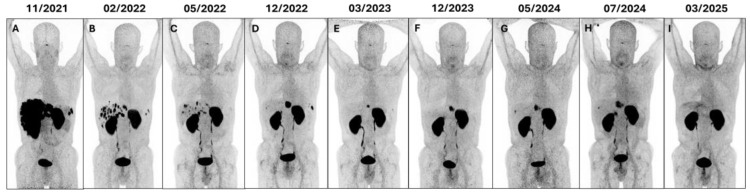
MIP sequence of [^68^Ga]Ga-DOTA-LM3 PET/CT scans of a 52-year-old patient diagnosed in 2014 with well-differentiated, functioning NEN of the pancreas G3 (Ki67 52%). He underwent pancreaticoduodenectomy and, due to hepatic and peritoneal lesions, received chemotherapy (2014), liver metastases resection (2018), 4 cycles of [^177^Lu]Lu-Oxodotreotide (Lutathera^®^, 2020), Sunitinib, Everolimus, Pembrolizumab, and somatostatin analogues (2021). Restaging with Gallium-68-labeled SSTR antagonist PET/CT ((A); 11/2021) showed massive progression with innumerable liver metastases and increased chromogranin A (>2000 µg/L), prompting two cycles of [^177^Lu]Lu-DOTA-LM3 PRRT (11/2021–02/2022), achieving partial remission (B,C). He also received immunotherapy with Nivolumab. Peritoneal recurrence in the upper abdomen led to two more cycles of [^177^Lu]Lu-DOTA-LM3 PRRT (12/2022–03/2023), resulting in minimal residual disease at [^68^Ga]Ga-DOTA-LM3 PET/CT (D,E). Subsequent [^68^Ga]Ga-DOTA-LM3 PET/CT scans (F–H) documented abdominal progression with increased chromogranin A (>8000 µg/L); therefore, he received [^177^Lu]Lu-DOTA-LM3 (08/2024) and [^225^Ac]Ac-DOTA-LM3 TANDEM-PRRT (10/2024), which was well tolerated. The patient benefited from PRRT, becoming physically active without any toxicity during follow-up; this allowed him to successfully undergo hepatic and diaphragmatic surgery in late 2024. [^68^Ga]Ga-DOTA-LM3 PET/CT in March 2025 (I) demonstrated complete remission, particularly in the para-gastric region and liver. As of April 2025, the patient is alive and in excellent clinical condition (having recently won a pickleball competition), 6 months after TANDEM-PRRT and 11 years after diagnosis.
